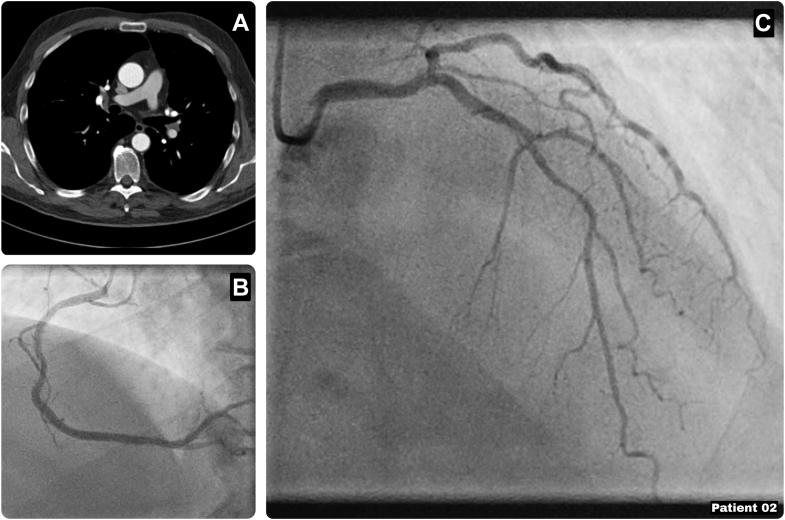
Characteristics of Patient 2 (A) Preoperative computed tomography demonstrating patent bilateral internal mammary arteries without aortic calcifications. (B and C) Coronary angiography showing a significant LAD stenosis (fractional flow reserve: 0.71), with nonsignificant lesions in the diagonal and circumflex artery and a patent RCA stent. LAD = left anterior descending artery; RCA = right coronary artery.
